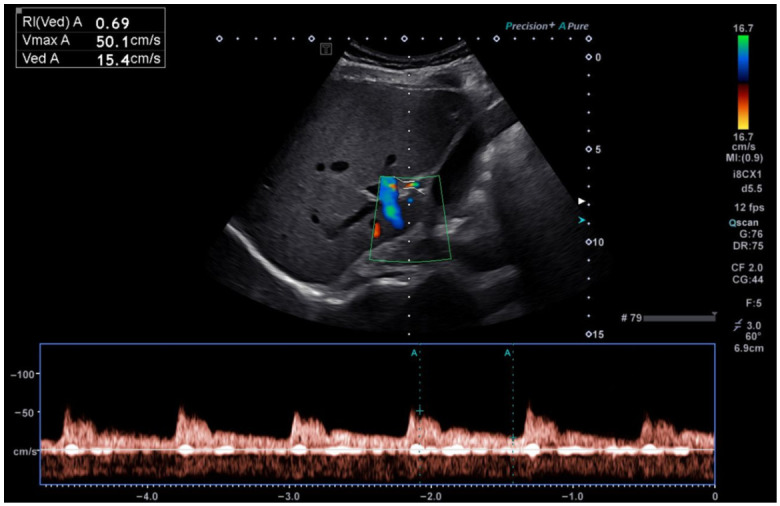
A color Doppler US evaluation for the same patient: in this case hepatic artery spectral analysis with normal flow parameters.
